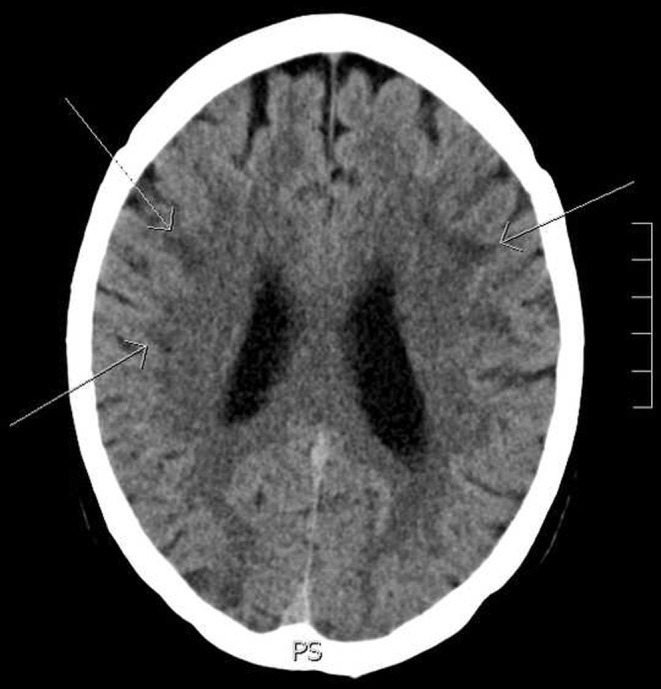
Computed tomography axial of the brain showing multiple hypodense subcortical areas suggestive of ischaemia
